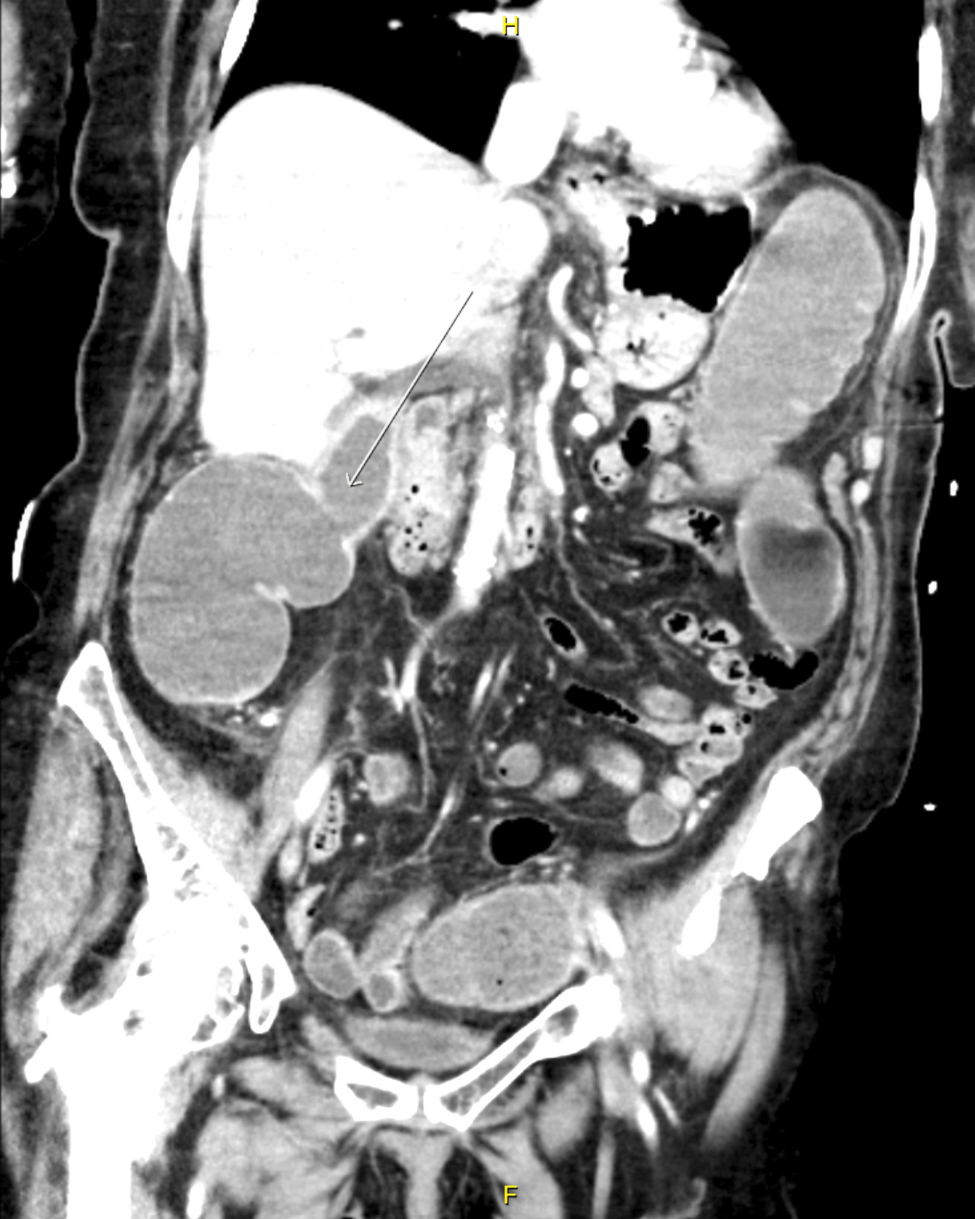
Abdominal CT scan: coronal view showing the cholecysto-colonic fistula connecting the gallbladder to the hepatic flexure (arrow)
